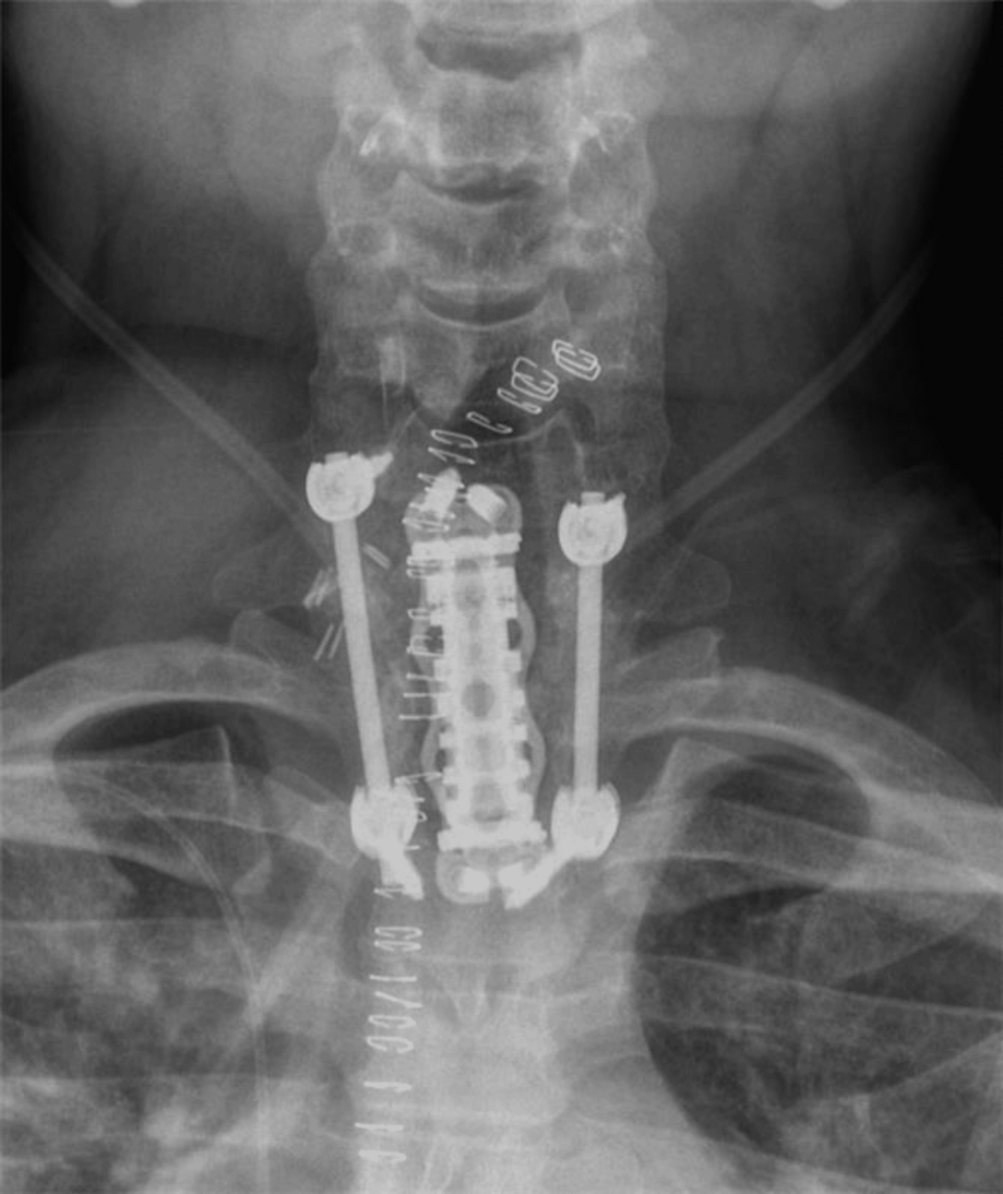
Post-operative CT spine demonstrating changes consistent with anterior cervical corpectomy and posterior cervical fusion
